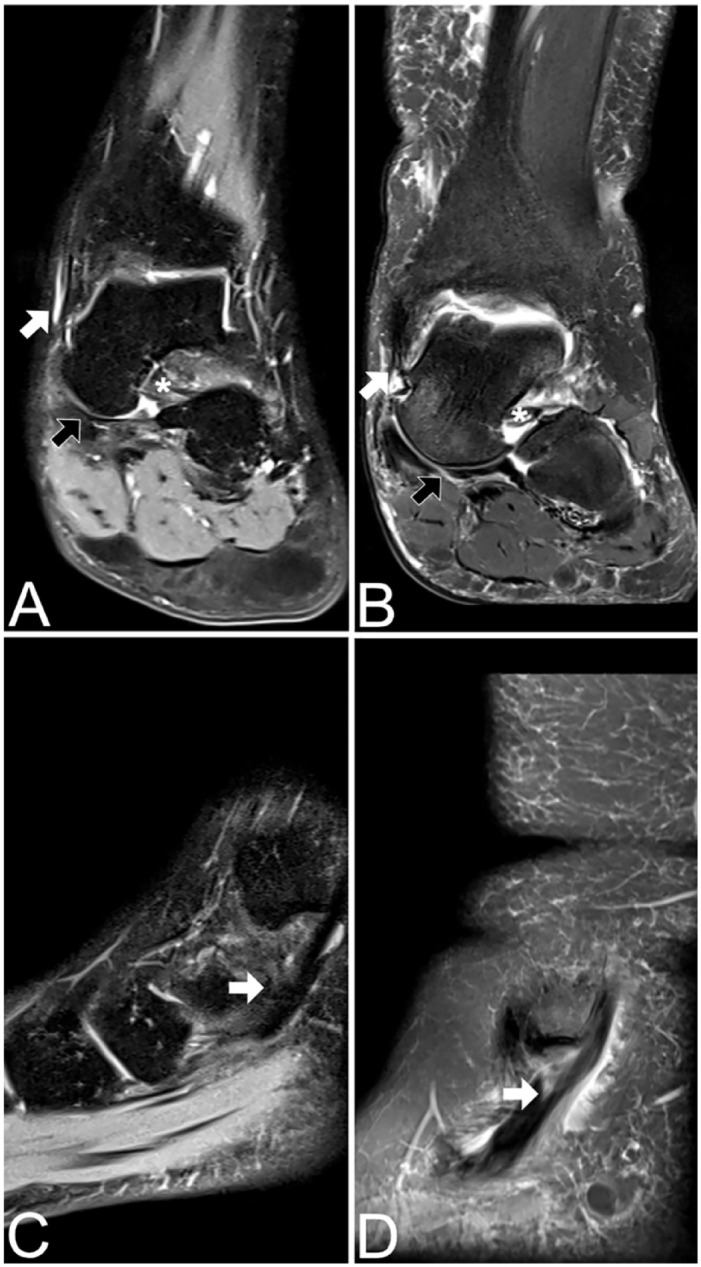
MR images showing tibiospring ligament, spring ligament, and tibialis posterior tendon in PCFD patients. (A) PD-weighted fat-saturated (FS) coronal image shows intact tibiospring (white arrow) and intact spring (black arrow) ligament. (B) PD-weighted FS coronal image shows complete tear with residual proximal fibers of the tibiospring ligament (white arrow) and a torn spring ligament (black arrow). (C) PD-weighted FS sagittal image shows intact tibialis posterior tendon. (D) PD-weighted FS sagittal image shows partial split-tear of the tibialis posterior tendon. PCFD, progressive collapsing foot deformity.
